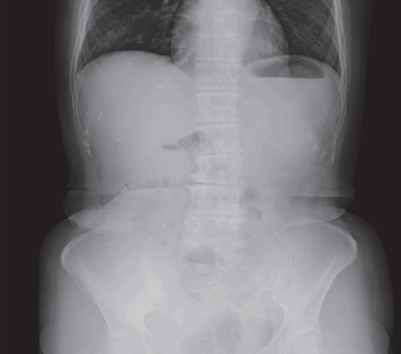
Abdominal plain radiograph of case 1 on admission showed a markedly dilated small bowel, with no dilatation of the colon.
